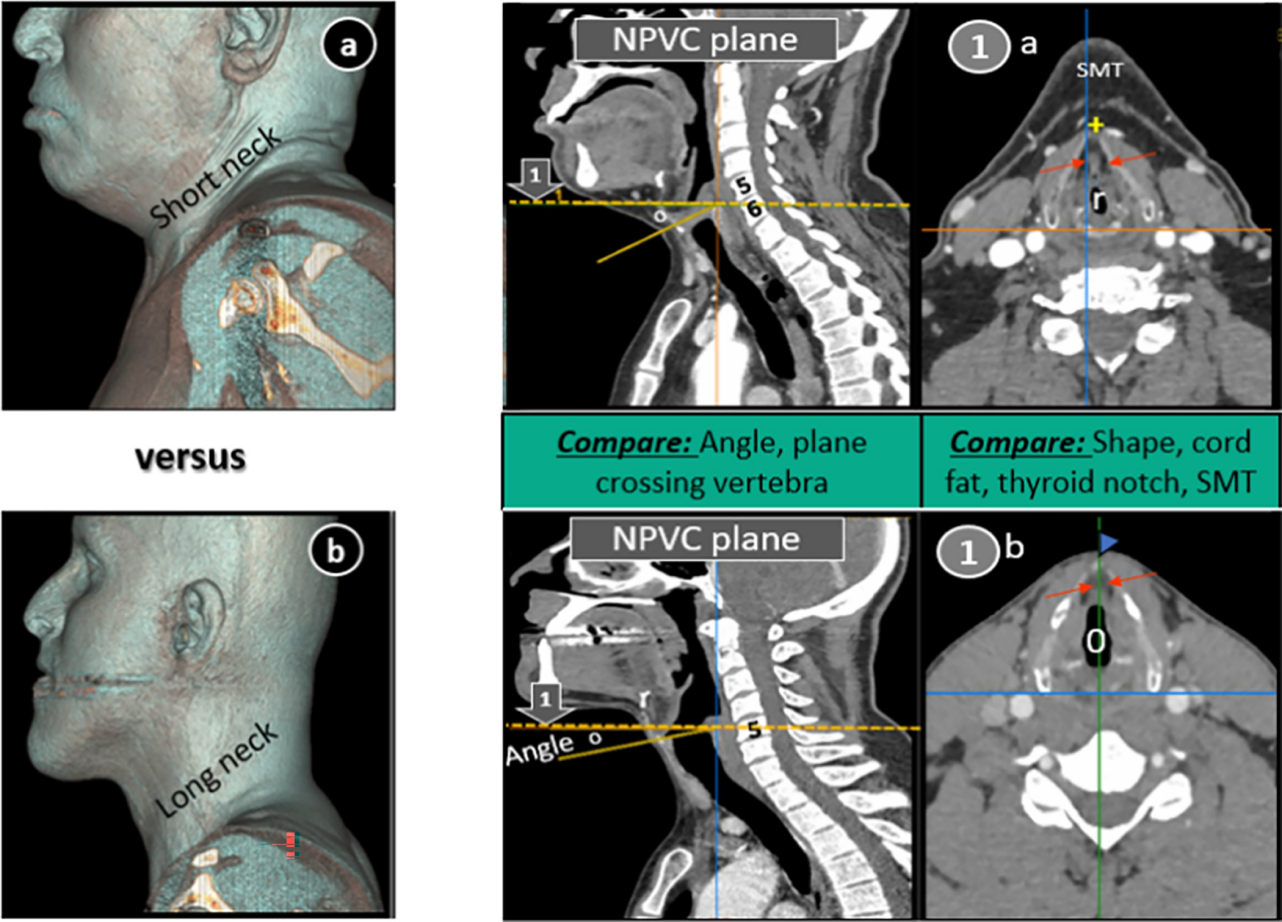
3D CT volumetric neck reconstruction of short versus long neck (a, b) along with comparison of their necks on sagittal and axial CT scans. Obliquity of non-parallel vocal cord (NPVC) plane (o) and variation of plane crossing vertebrae are shown in sagittal sections indicating more in short neck. Visualisation of structures which are not part of glottis are seen more in short neck (1a) than long neck (1b). Different shapes (round, r/oval, 0) affect quantitative parameters as well.
